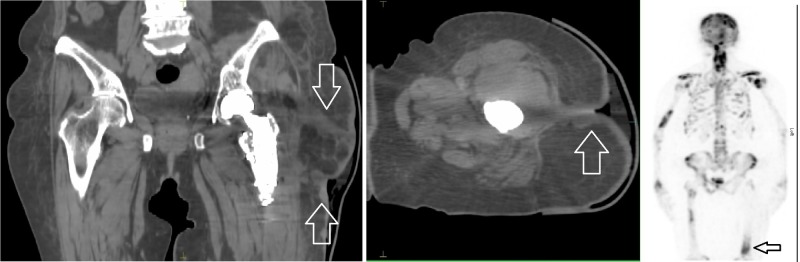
Coronal (left) and axial (centre) computed tomography (CT) scan images showing area of recurrence tracking along surgical scar site (white arrows) and bone scan (right) showing distal femur progression below previous radiation field (black arrow).
